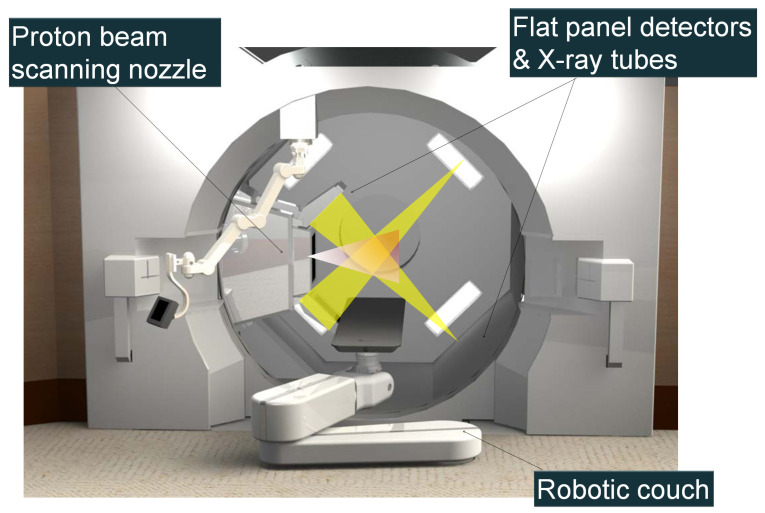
Hitachi RGPT Hitachi RGPT system on a rotating gantry. The horizontal orange beam represents the proton particle beam. The two mutually orthogonal yellow beams represent the on-treatment kV fluoroscopic imaging beams used for real-time fiducial-marker tracking; the corresponding kV X-ray tubes and flat-panel detectors are mounted directly on the gantry so that the imaging geometry rotates with the treatment beam. Reproduced from Shimizu et al. [76] under the terms of the Creative Commons Attribution License (CC BY 4.0).
